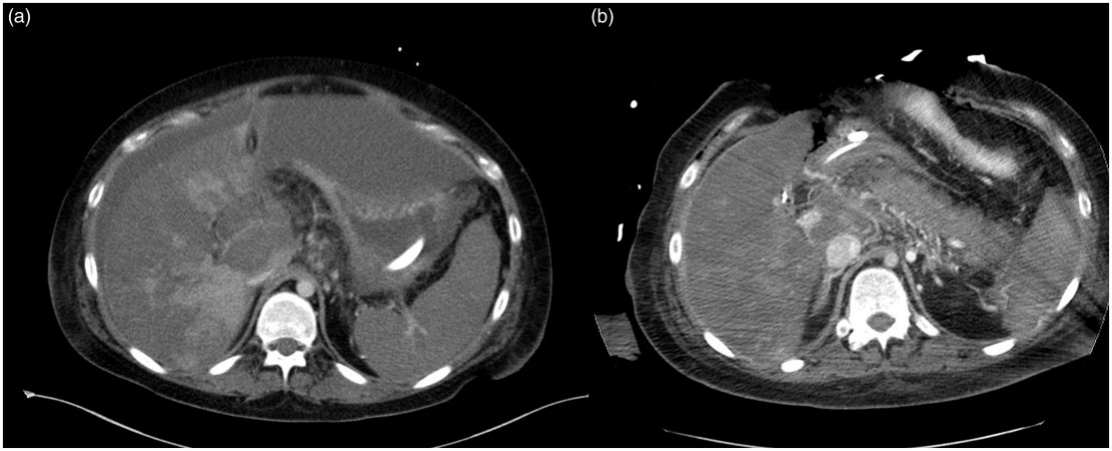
(a) Axial CT images showing extensive perfusion deficit of the liver
